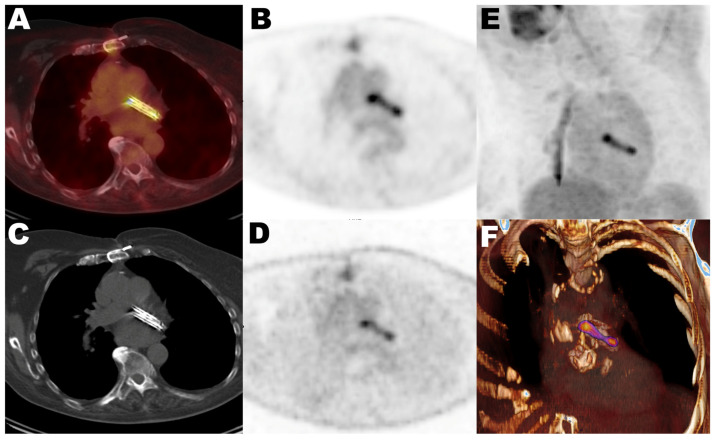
Representative case showing radiotracer uptake in axial images of FDG-PET/CT (A), attenuation-correction FDG-PET (B), CT (C) and non-attenuation-correction FDG-PET (D), as well 3D volumetric reconstruction of FDG-PET (E) and hybrid FDG-PET/CT (F). The most common uptake pattern observed across positive studies was characterized by homogeneous radiotracer activity related to the LAACD, with more prominent activity at the extremities of the device.
